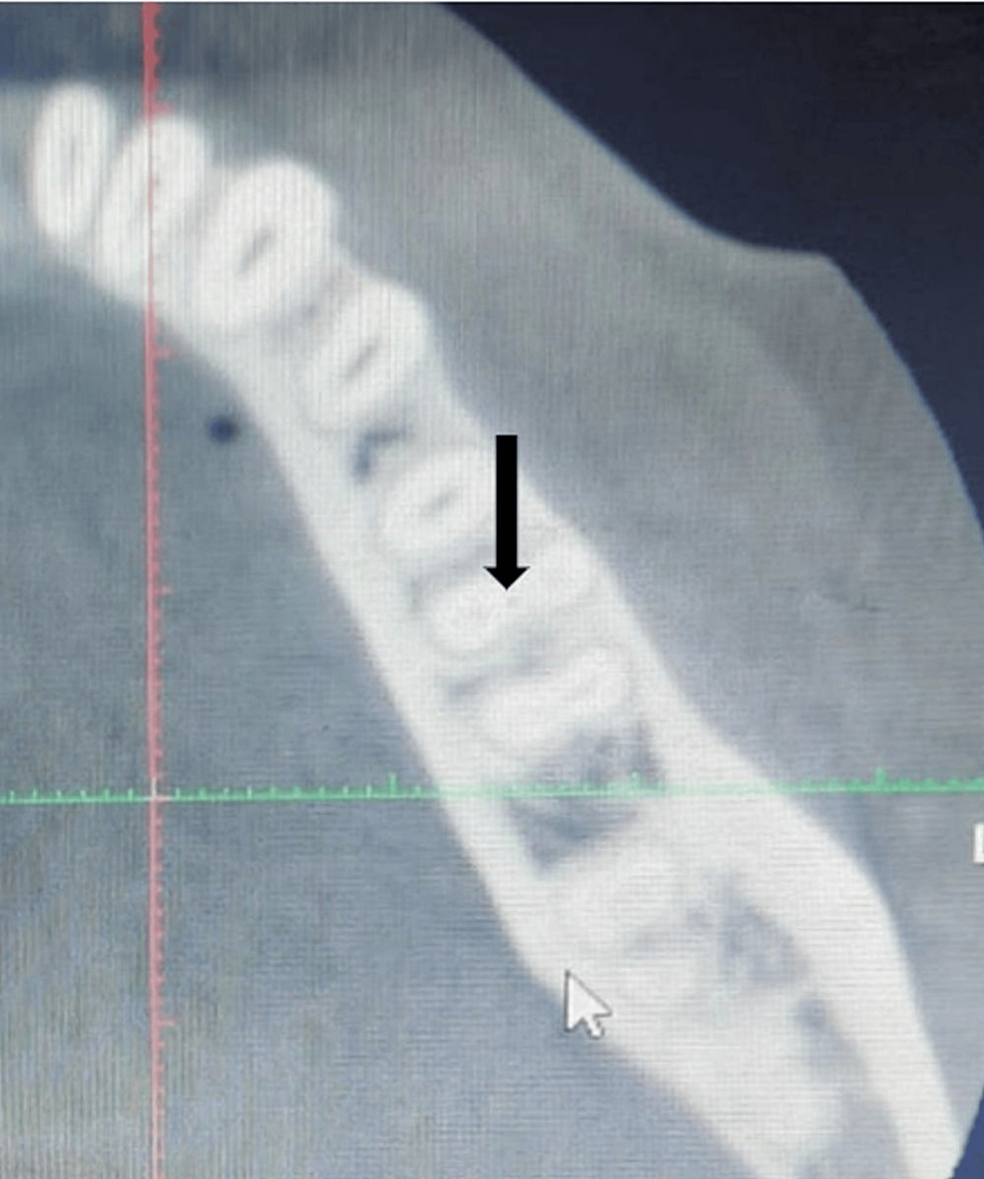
Middle Mesial Canal The black arrow denotes the cone-beam computerized tomographic image showing middle mesial canal with separate canal orifice in axial view.
